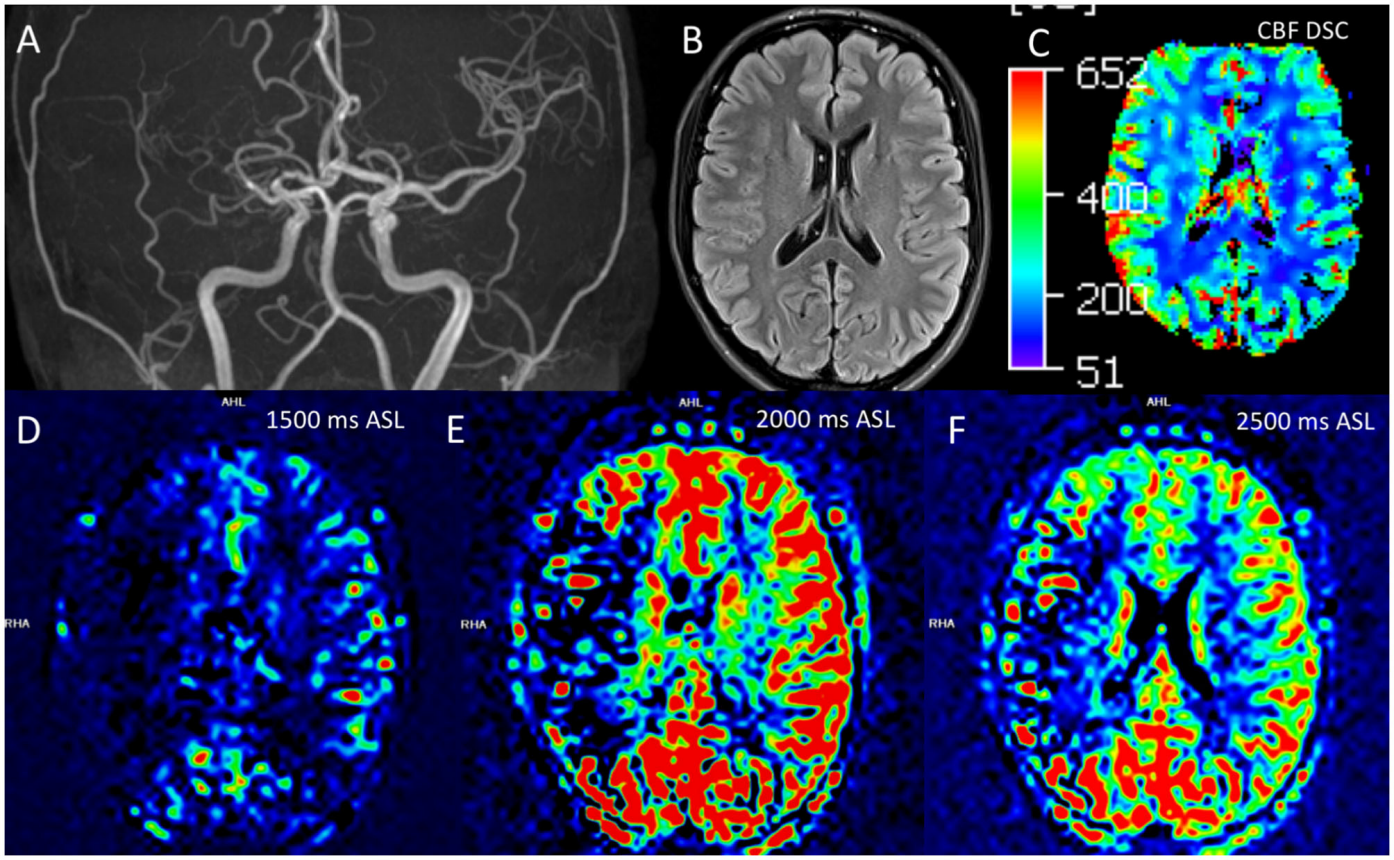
Comparison of ASL and DSC assessments of perfusion in moyamoya disease. Correct interpretation of ASL is heavily dependent on knowledge of the technical parameters and underlying basis for signal generation. A 21 year-old-female had findings of right sided MMD, including stenosis of the right M1, M2, and A1 segments on this coronal 3D-TOF MIP (A). Routine cross-sectional images such as this representative 2D T2 FLAIR image did not demonstrate chronic changes, including lack of infarct, white matter T2 hyperintensity, or Ivy sign (B). DSC perfusion shows preserved or slightly increased CBF in the right cerebral hemisphere (C). ASL perfusion was also obtained with multiple tag delay time points including 1,500 ms (D), 2,000 ms (E), and 2,500 ms (F). The ASL images suggest decreased flow in the right MCA territory; this appearance is more marked at the 2,000 ms timepoint. However, this low ASL signal represented delayed transit through collateral pathways rather than low CBF, demonstrating the ASL must be interpreted in context of the technique and pathophysiology. ASL, arterial spin labeling; CBF, cerebral blood flow; DSC, dynamic susceptibility contrast; FLAIR, fluid-attenuated inversion recovery; MIP, maximal intensity projection; TOF, time of flight.
